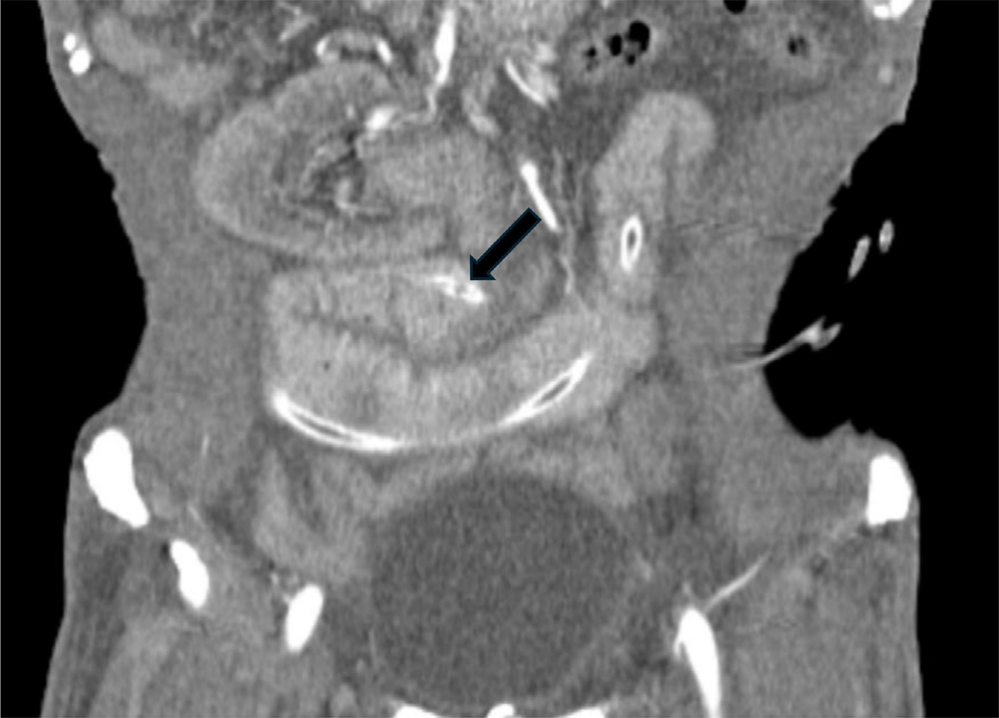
Coronal abdominal CT reconstructions showing intestinal intussusception around a feeding jejunostomy tube (black arrow).
